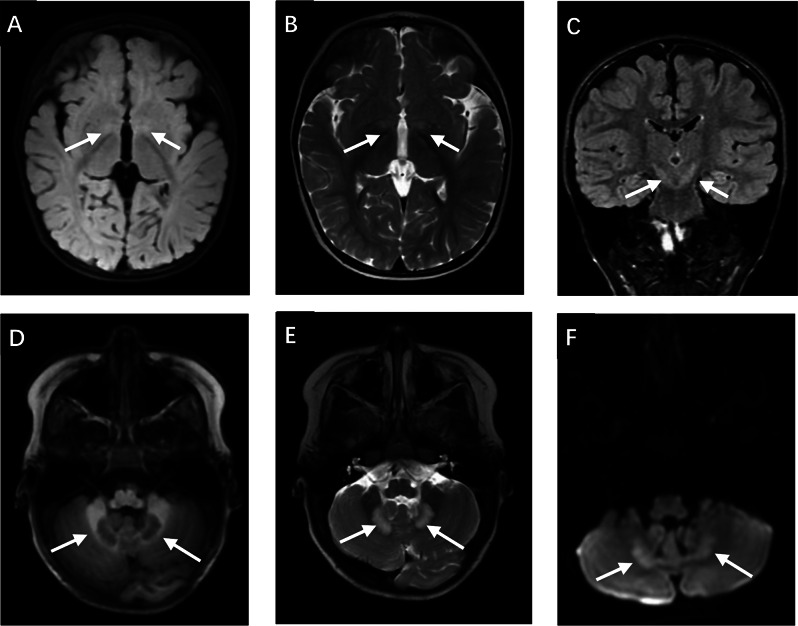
Brain MRI of Patient 4 (10 months old) showed symmetric T2 and T2-FLAIR long abnormal signals in both sides of the pallidus (A, B, C, the white arrow) and cerebellar hemispheres (D, E, F, the white arrow)
